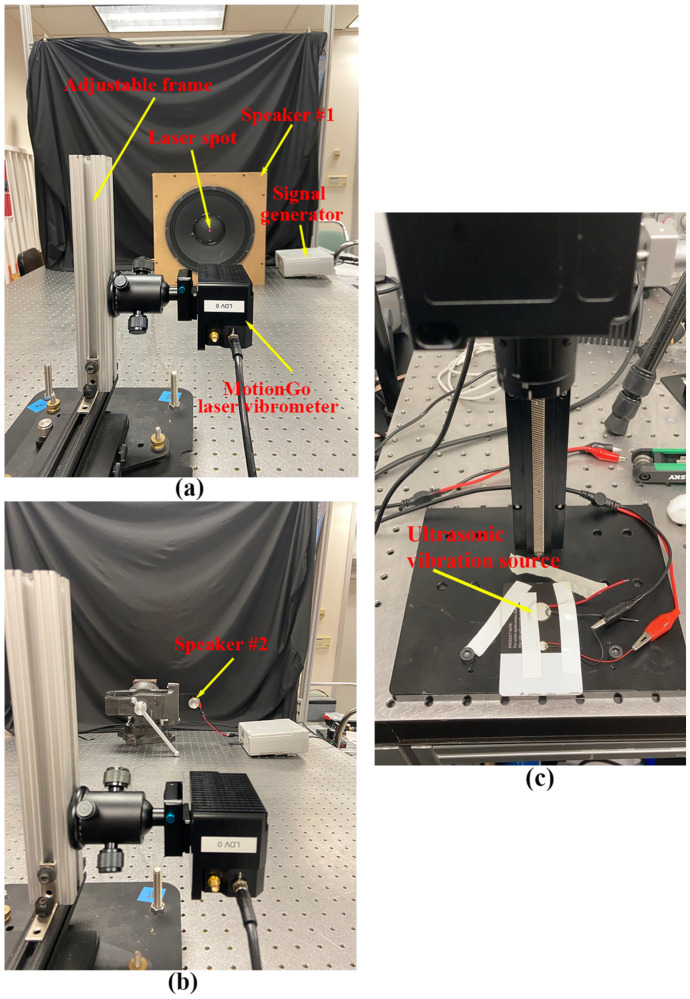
Experimental setups of the measurement repeatability validation of the proposed laser vibrometer: (a) a speaker under excitation frequencies of 0.1 Hz–1000 Hz; (b) another speaker under excitation frequencies of 5000 Hz and 20,000 Hz; and (c) an ultrasonic vibration source under an excitation frequency of 1,000,000 Hz.
